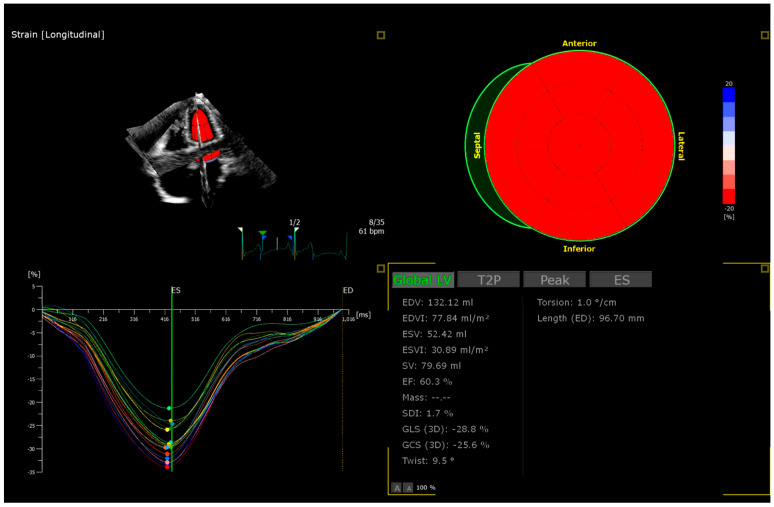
Figure to show 3D echocardiography assessment—LV-GLS-3D.
